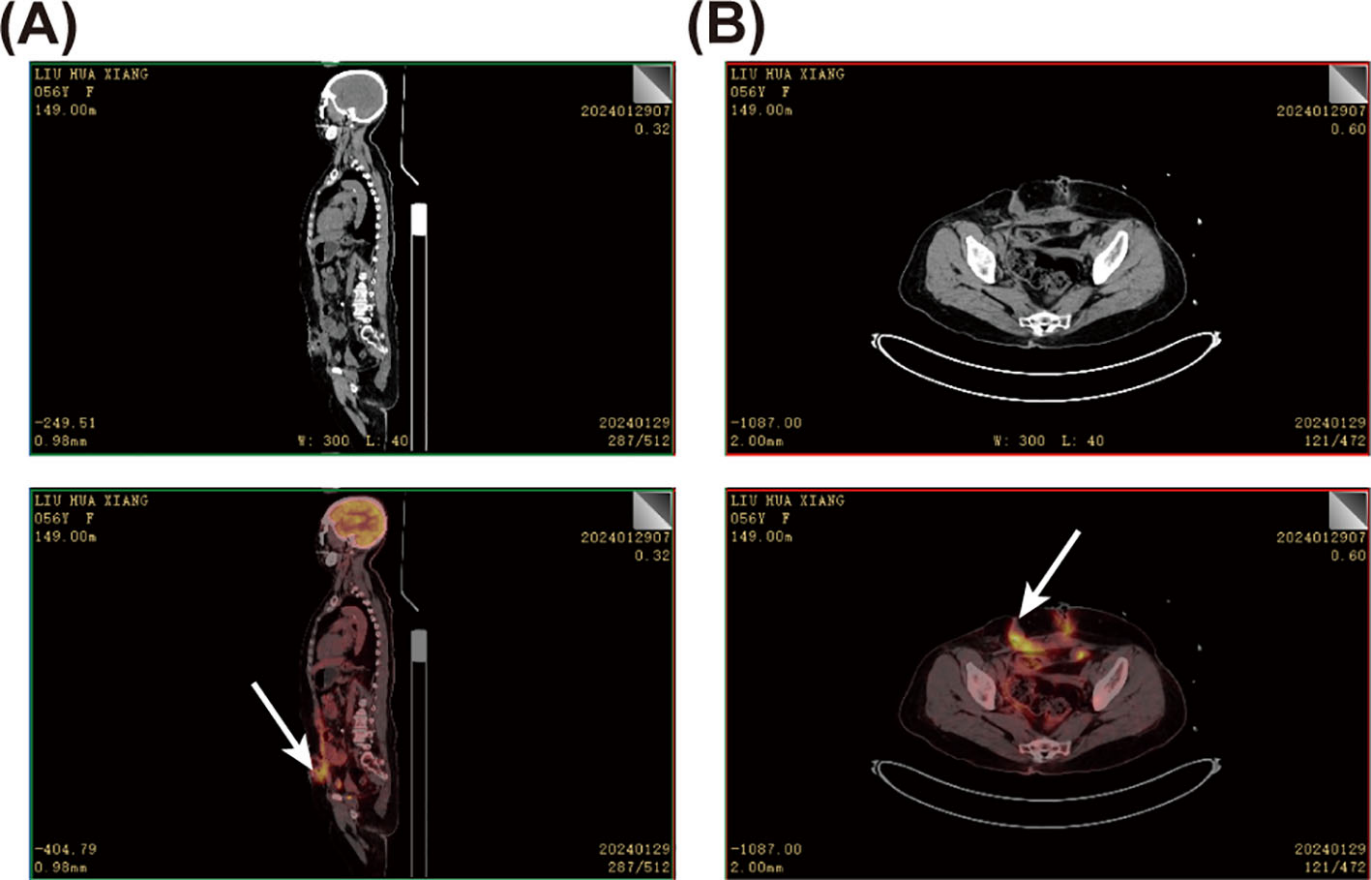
Positron emission tomography-computed tomography (PET-CT) of the patient. (A) Sagittal PET-CT image of the patient. (B) PET-CT of the abdomen. The white arrow points to the lesion with a high standard uptake value (SUV) uptake, suggesting a high probability of a tumor.
